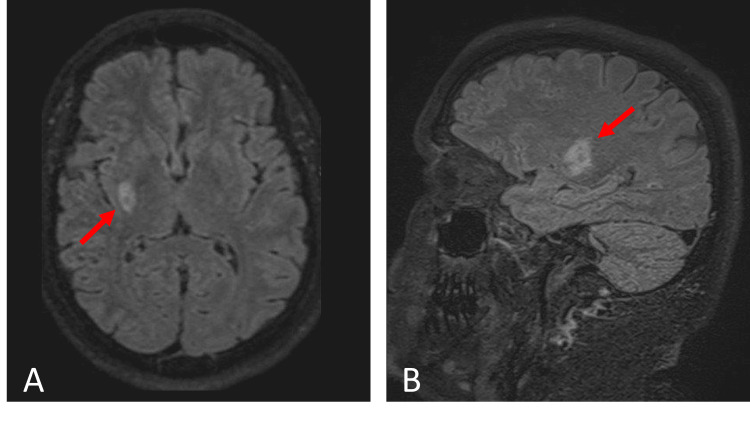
MRI one month later revealing a FLAIR hyperintensity (red arrows) consistent with right striatocapsular infarction.
